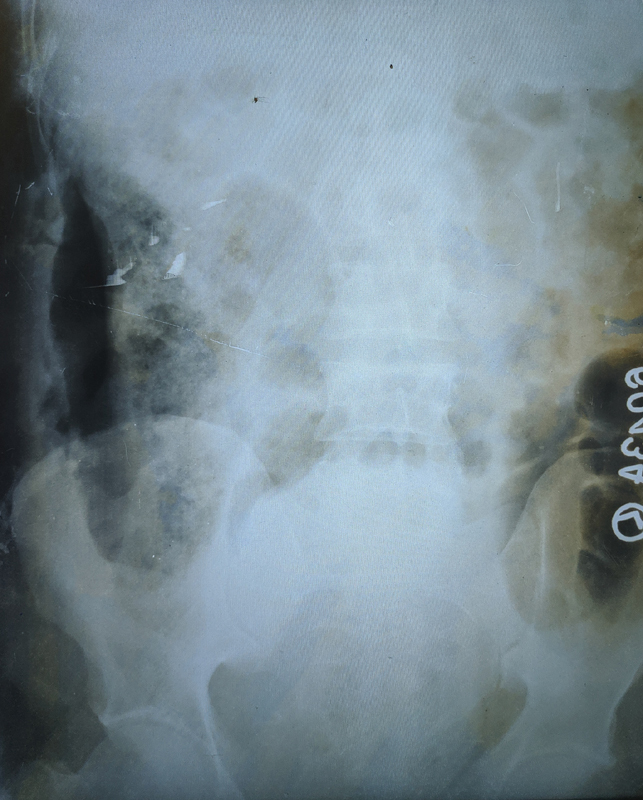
X-ray abdomen in standing position showing radiolucency in the right lateral abdomen.
